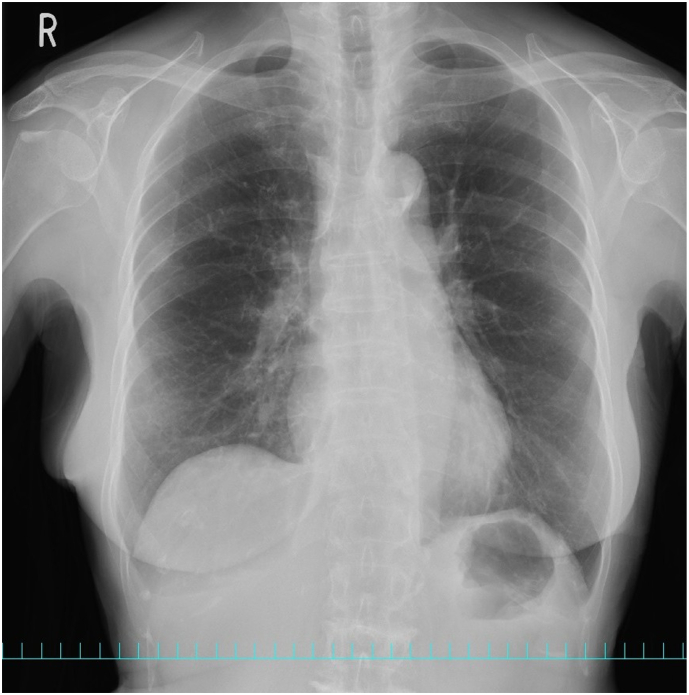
Chest roentgenogram 1 year after the operation showing no apparent tumor shadow, and pleural effusion.
